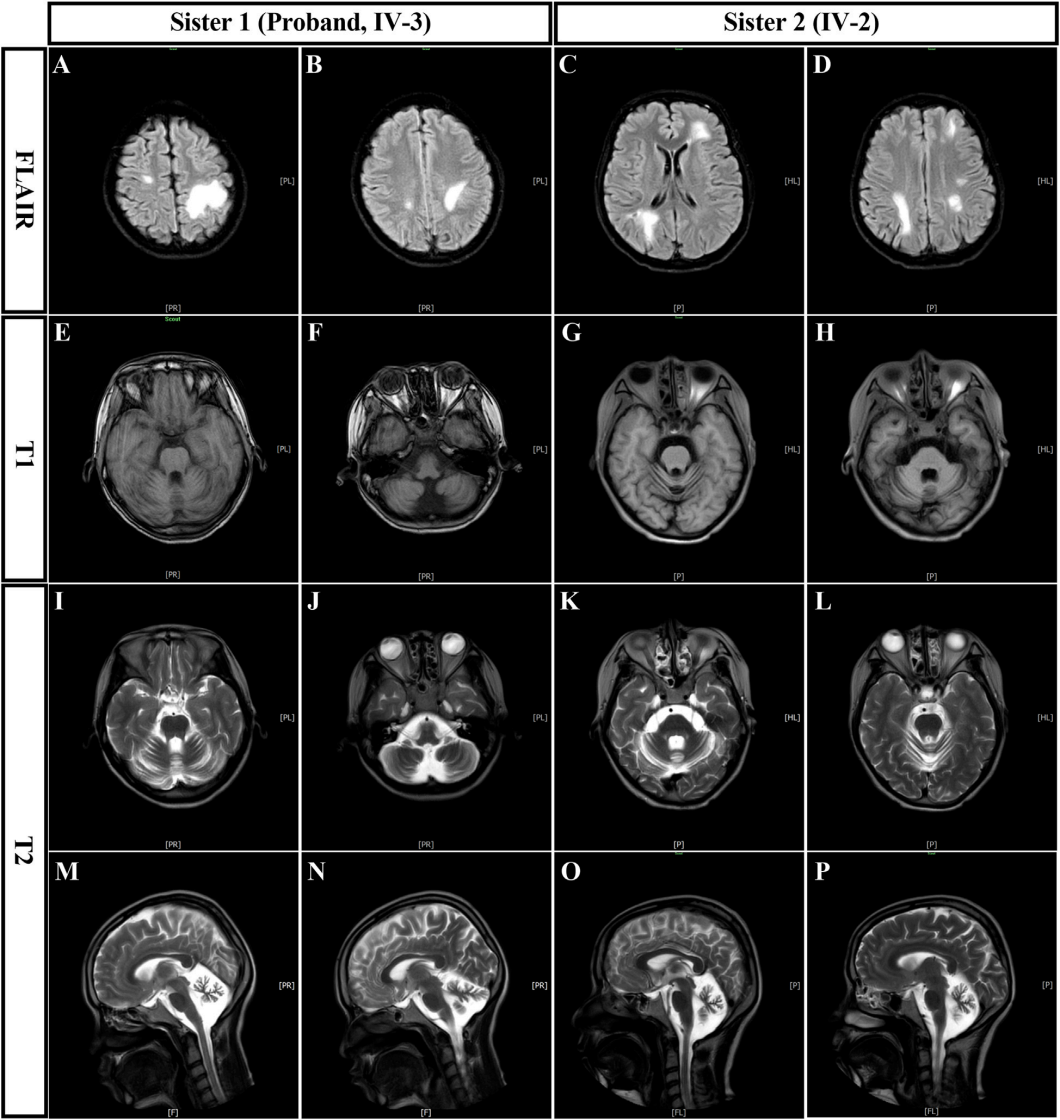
Representative cranial MRI of the affected sisters with Ataxia-Telangiectasia. (A, B): The FLAIR sequences for Sister 1 (IV-3) displayed patchy high-signal lesions in the right frontotemporal lobe and left parietal lobe, suggesting white matter abnormalities. (C, D): The FLAIR sequences for Sister 2 (IV-2) also demonstrated similar patchy high-signal lesions in the left frontal lobe and bilateral parietal lobes. (E, F): The T1-weighted images for Sister 1 revealed mildly low signals, with no obvious abnormalities in the brainstem or cerebellum. (G, H): The T1-weighted images for Sister 2 showed an isointense signal. (I, J): The T2-weighted axial images for Sister 1 showed a high signal. (K, L): The T2-weighted axial images for Sister 2 demonstrated a high signal. (M, N): The sagittal T2-weighted images for Sister 1 showed deepened cerebellar sulci, indicating cerebellar atrophy. (O, P): The sagittal T2-weighted images for Sister 2 revealed similar findings of cerebellar atrophy, confirming structural degeneration. MRI, cranial magnetic resonance imaging.
